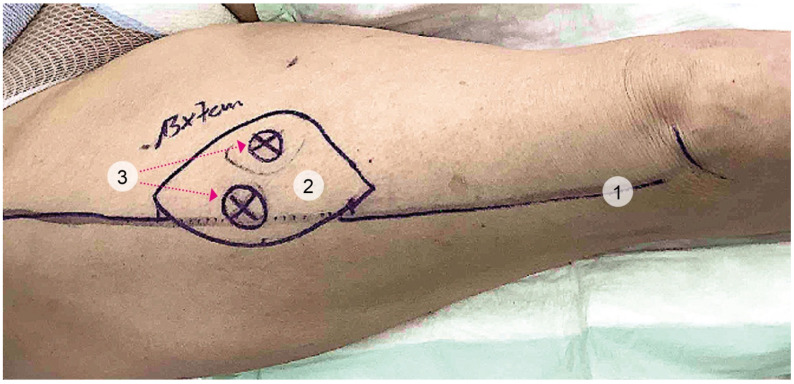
Preoperative markings. 1: line from superior anterior iliac spine to lateral margin of patella. 2: size-matched ALT. 3: Doppler-located perforators.
